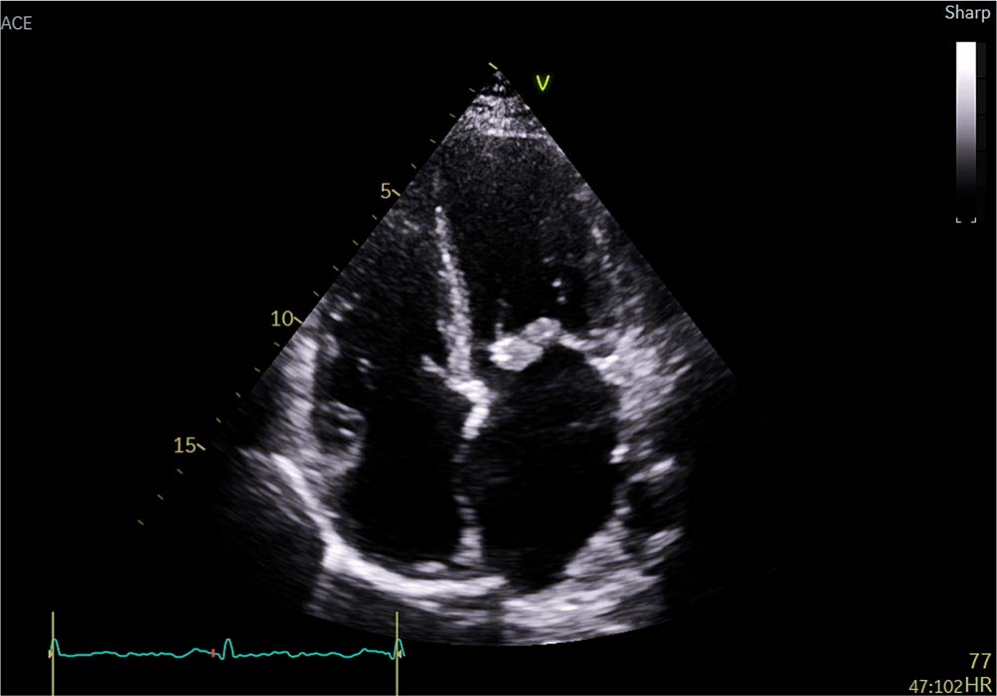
Transthoracic echo appearance of mitral valve with mass attached to posterior leaflet (apical 4 chamber view).
